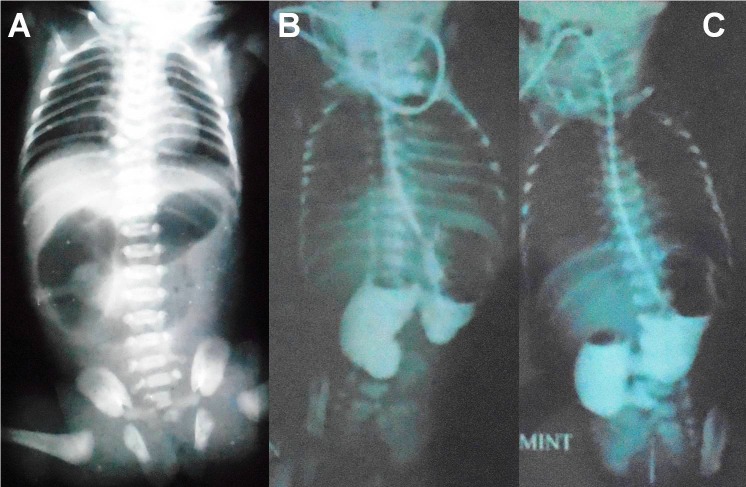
Figure 1: Abdominal X-ray showing gastric and duodenal gas (C-shape) shadow extending beyond the second part of duodenum along with absence of distal air (A); upper gastro-intestinal contrast study suggesting obstruction at the third part of the duodenum (B,C).
